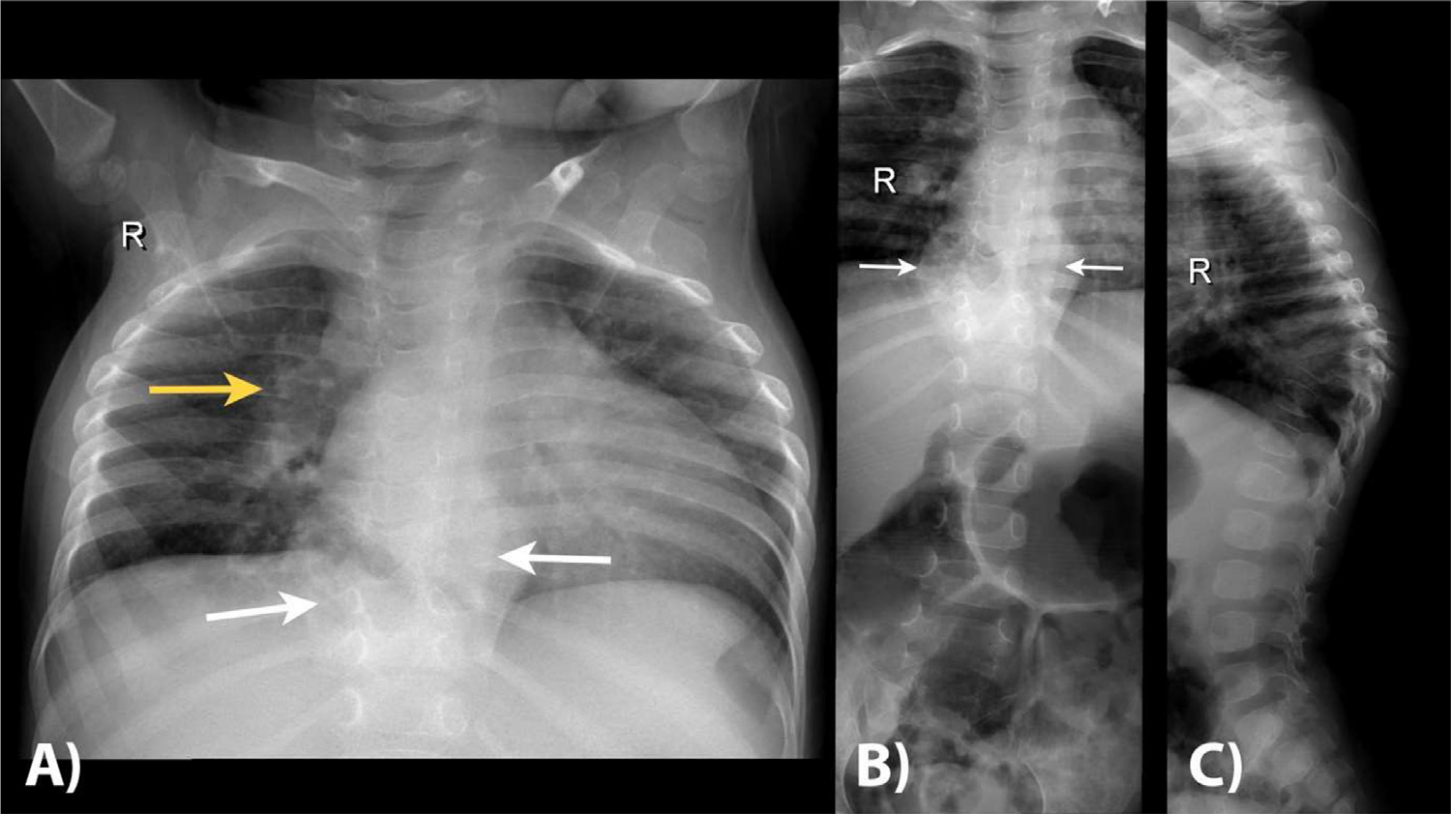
A conventional radiograph of the thorax showed right perihilar pulmonary consolidation (yellow arrow in A), Gibbus formation at the level of T8-10 and soft tissue mass appearance at the level of T5-11 (white arrows on A). The thoracolumbar radiograph confirmed the presence of vertebral bodies destruction and paravertebral soft tissue mass between the T5-11, suggesting the diagnosis of tuberculous spondylitis with paravertebral abscess (arrows in B and C).
